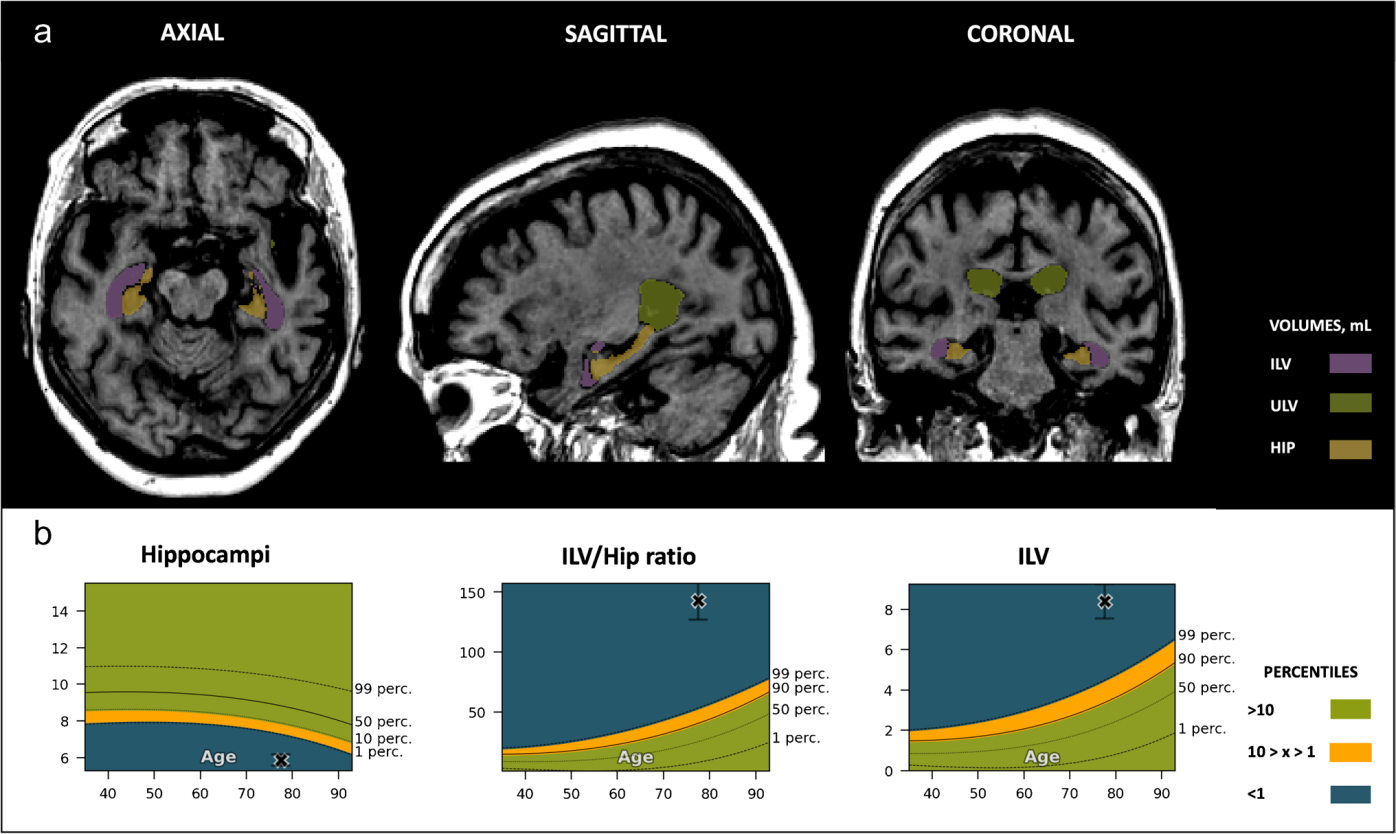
Dementia (DEM) case. Cross-sectional coronal T1-weighted image at the level of the medial temporal lobe and hippocampus with segmentation of the upper lateral ventricles (green), inferior lateral ventricle (purple), and hippocampus (yellow). A — Automated volumetric measurements *left*: axial view; *middle*: sagittal view; *right*: coronal view. B — Population graphs *left*: hippocampal volumes of this subject showing the gray “x” marker in the blue region, below the 1st percentile; *middle*: the ILV/Hip ratio of this subject showing the gray “x” marker in the blue region, above the 99th percentile. *Right*: ILV volumes of this subject showing the gray “x” marker in the blue region, above the 99th percentile. Color-coded population graph percentile interpretation; 1–90th percentiles, green (normal); 90–99th percentiles, orange (caution); > 99th percentile, dark blue (abnormality)
